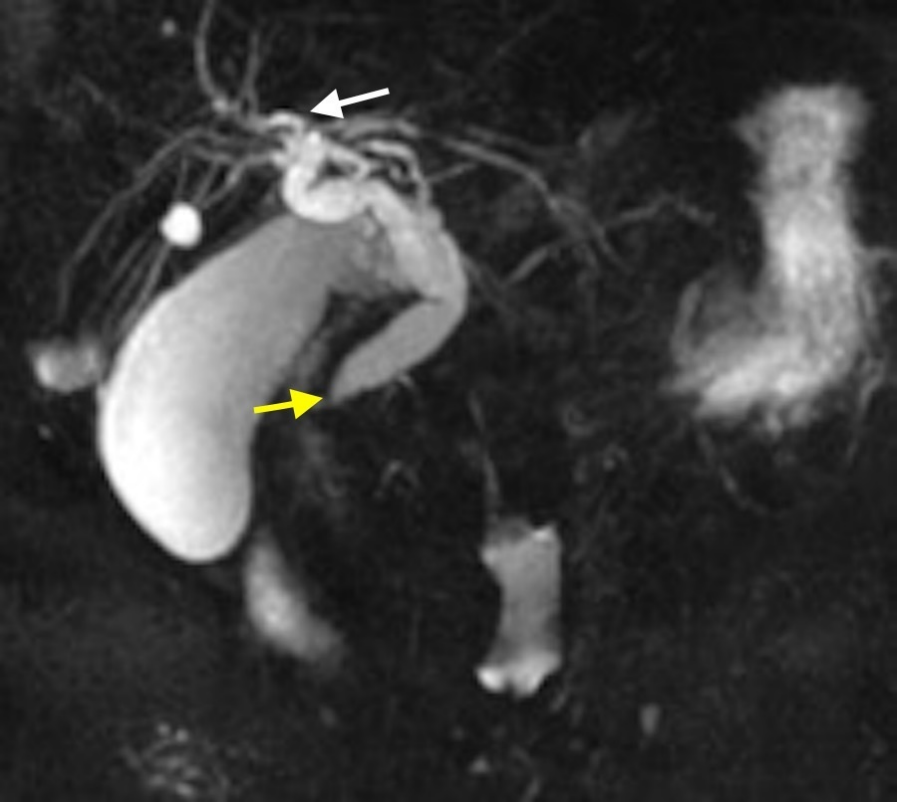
MRI showing dilated biliary ducts (white arrow) with tapering of the distal common bile duct (yellow arrow)
